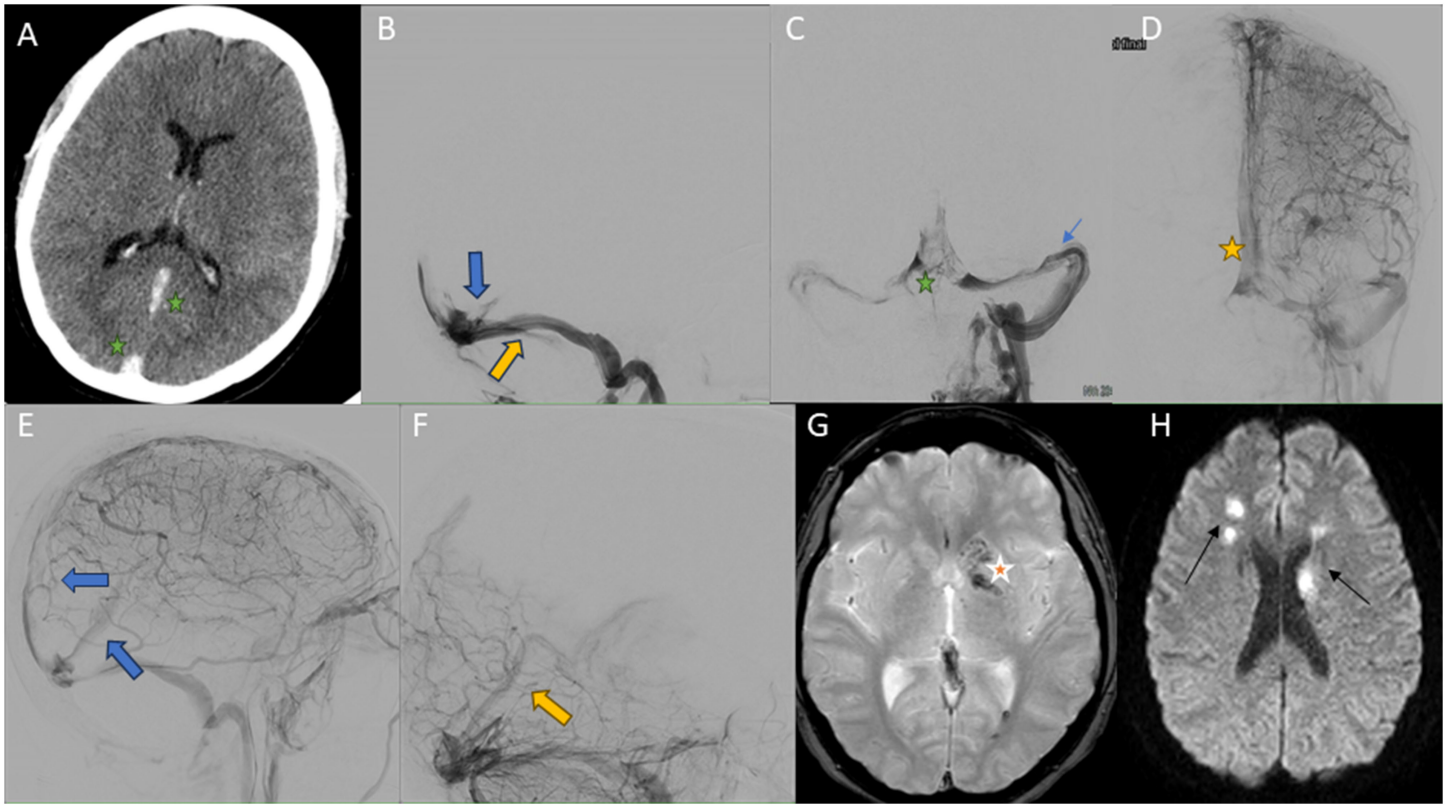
Images obtained during the course of case 1. (A) Cerebral CT scan without intravenous contrast. CVT with hyperdensity of superior sagittal sinuses (green star), rectus sinus (green star), vein of Galen, and internal cerebral veins. (B) Cerebral venography obtained by left internal jugular access, lateral view. An extensive thrombus with involvement of the rectus sinus is visualized (blue arrow). Direct aspiration was performed with the Neuron MAX 0.88^®^ device (yellow arrow), followed by access to the rectal sinus with a microcatheter and TigerXL^®^ device (not shown in the image). (C) Cerebral venography obtained by left internal jugular access, anteroposterior view. Recanalization of left transverse-sigmoid sinuses (green star) is evident after manual and pump aspiration through Neuron MAX 0.88^®^ and JET7^®^. Guide catheter is can be seen in these sinuses (blue arrow). (D, E) Selective left internal carotid arteriography cerebral arteriography in venous phase, anteroposterior (D, E) view. Repermeabilization of the previously occluded sinuses can be seen (superior sagittal sinus [yellow star in (D) and blue arrow in (E)], rectus sinus and deep cerebral veins [blue arrow in (E)]), with residual partial thrombosis persisting at the torcula. (F) Selective left vertebral arteriography lateral view. Recovery of deep venous drainage (yellow arrow). (G, H) Brain MRI, susceptibility-weighted (G) and diffusion weighted images (H). Multiple signal alterations with diffusion restriction affecting bilateral basal ganglia, right thalamus and supratentorial white matter, compatible with venous infarcts (black arrows) with hemorrhagic transformation (red star).
